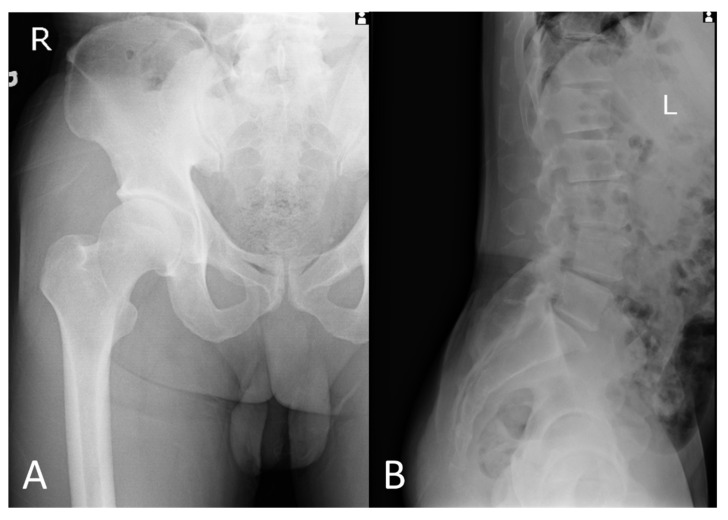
Plain radiographs of hip and lumbar spine. (A) Plain radiographs showed a hip with subtle subchondral bone sclerosis, which is compatible with early-stage OA. (B) Plain radiographs showed lumbar spines with spondylosis, disc space narrowing, degenerative sclerotic change at the L2/3 level, retrolisthesis at the L2/3, L3/4 level, and grade I spondylolisthesis at the L4/5 level, however not related to this acute pain.
